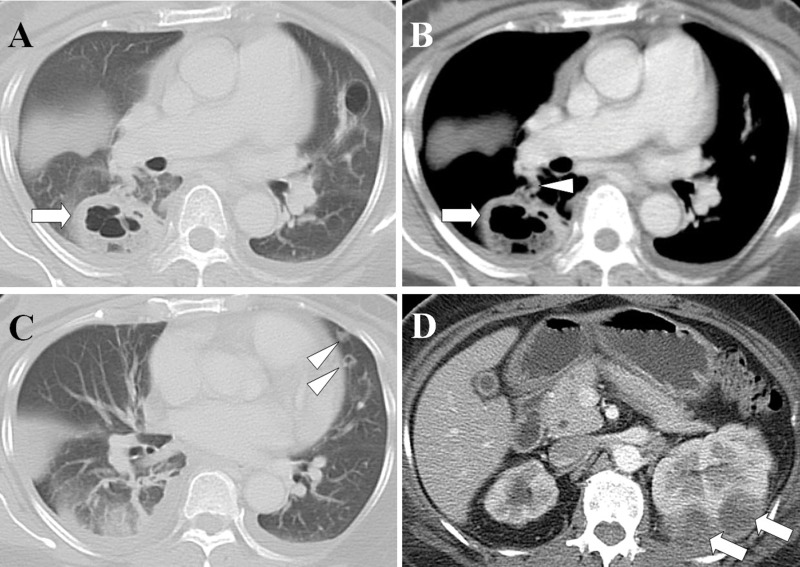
Renal abscesses with septic pulmonary emboli. (A) A lung window of a cross-sectional CT scan shows a lung abscess with a diameter of 4.5 cm in the right lower lobe (arrow). (B) A contrast-enhanced CT scan (mediastinum window) in the same image plane shows a lung abscess (arrow) with a feeding vessel sign (arrowhead). (C) A lung window of a cross-sectional CT scan shows two nodules with cavities in the left upper lobe (arrowheads). (D) An abdominal CT scan shows left renal abscesses (arrows). The patient was a 52-year-old woman whose blood cultures were positive for *Escherichia coli*.
